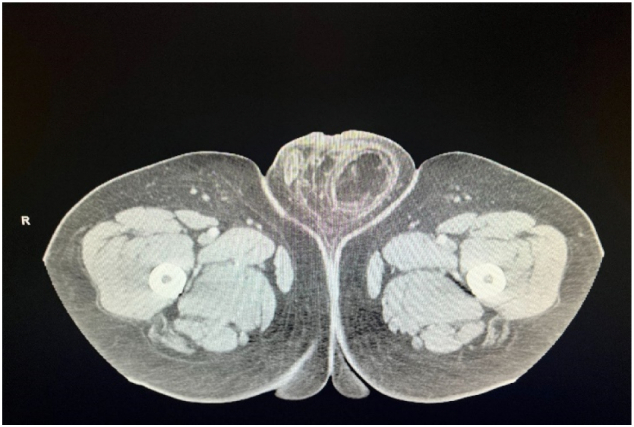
Axial image CT scan with what appears to be a large left fat filled hemiscrotum.
